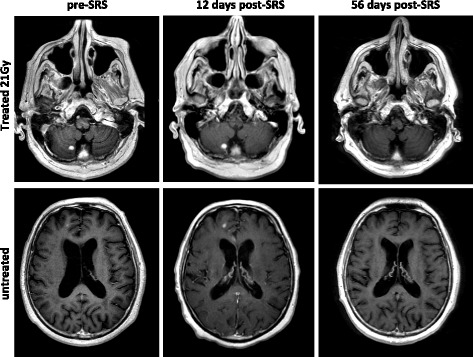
Untreated lesion response after SRS and pembrolizumab. In a patient who underwent SRS to a right cerebellar melanoma brain metastasis, a CR in an untreated right frontal lesion was observed. The right frontal lesion was not initially treated, as it was not clearly pathologic on pre-SRS scan. In an unplanned MRI 12 days post-SRS, the untreated lesion is more convincing of a metastasis. On planned follow-up MRI, both treated and untreated lesion display a CR
